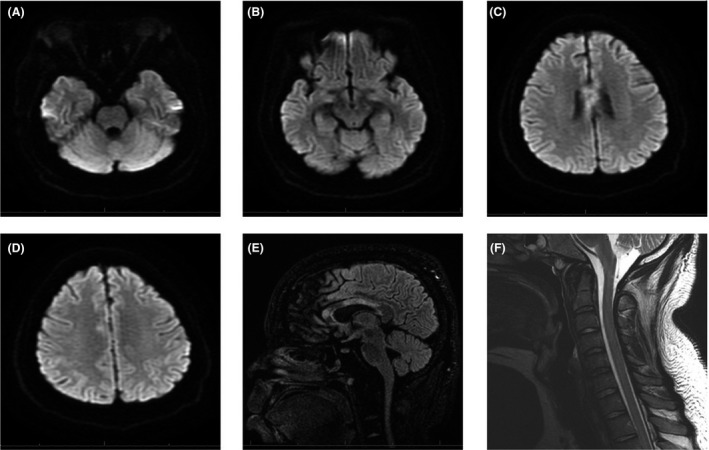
MRI images without gadolinium showing decreased signals in comparison with Fig. 1.
